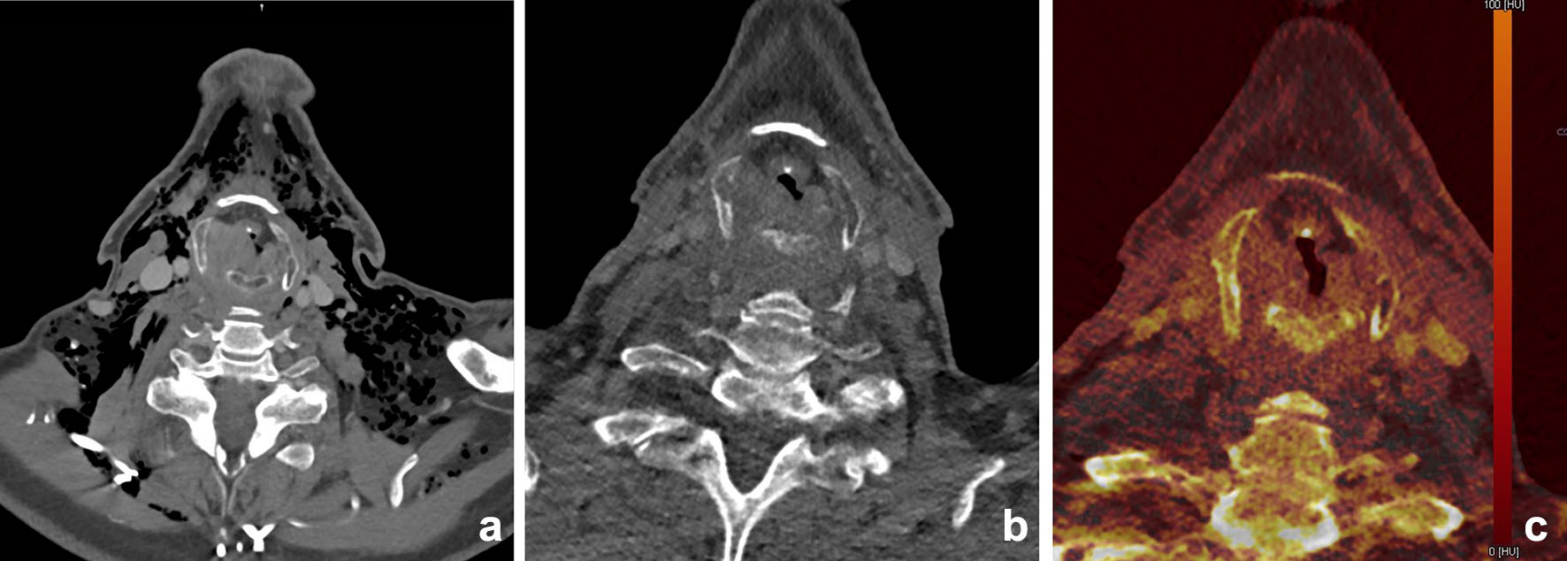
An 81-year old male patient with a hypopharyngeal carcinoma from the piriform sinus, presented 6 months after chemoradiation to evaluate therapy response. The initial CT with the presence of a bilateral tumor of the piriform sinus and massive (postoperative) subcutaneous emphysema (a). A mixed DECT at one year (b) was made for evaluation of therapy effect and exclusion of residual tumor. Bilateral swelling is present, without clear enhancement at mixed imaging. At the iodine overlay images (c), no increased iodine uptake was present; benign posttreatment changes without residual or recurrent tumor were concluded
